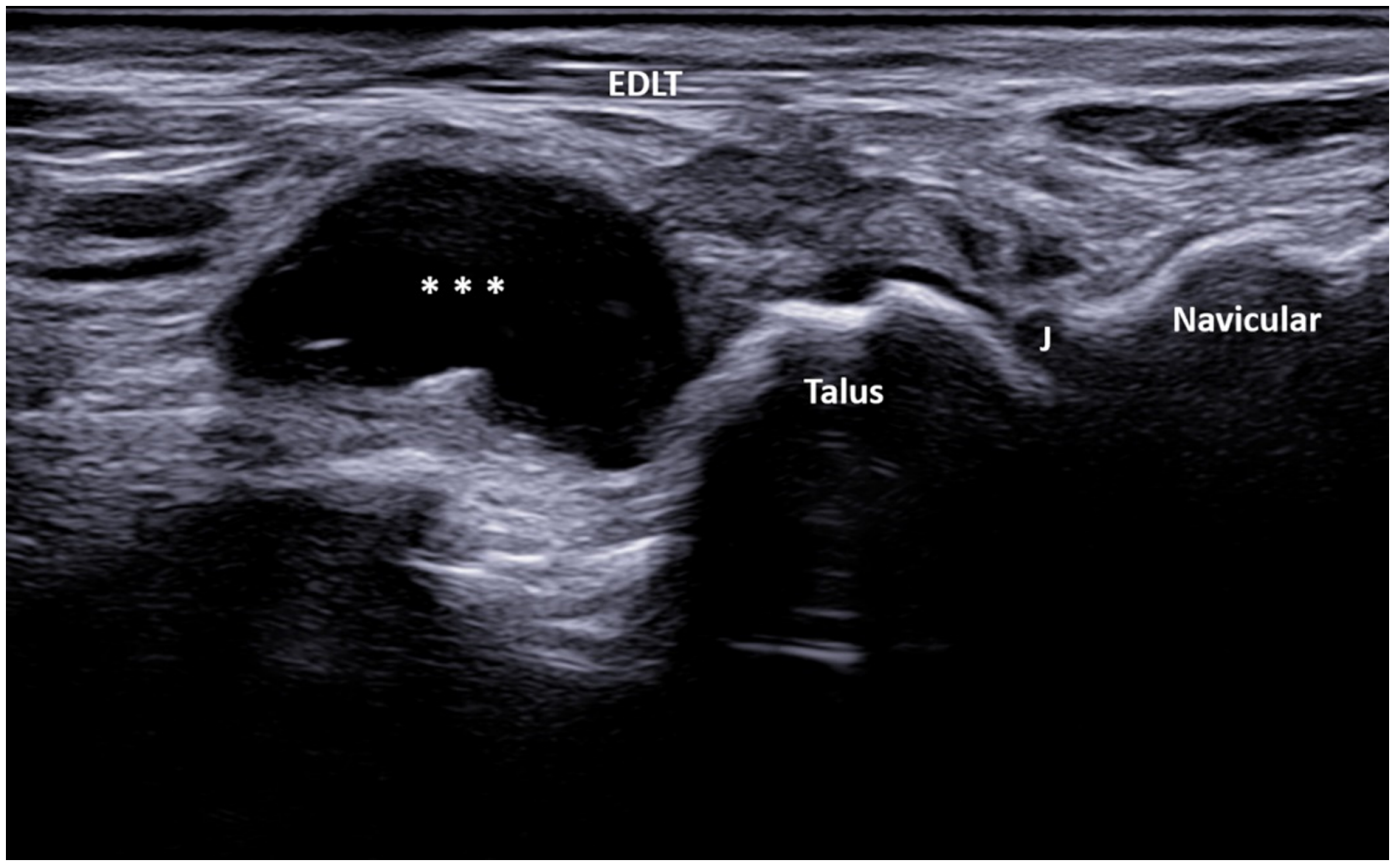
A 67-year-old patient with osteoarthritis. Ultrasound image showing GB with the transducer positioned in the long axis of the foot (left side is proximal). The longitudinal ultrasound image of the dorsolateral ankle reveals an anechoic unilocular fluid collection (*) between the extensor digitorum longus tendon (EDLT) and the talus. J, talonavicular joint and EDLT, extensor digitorum longus tendon.
